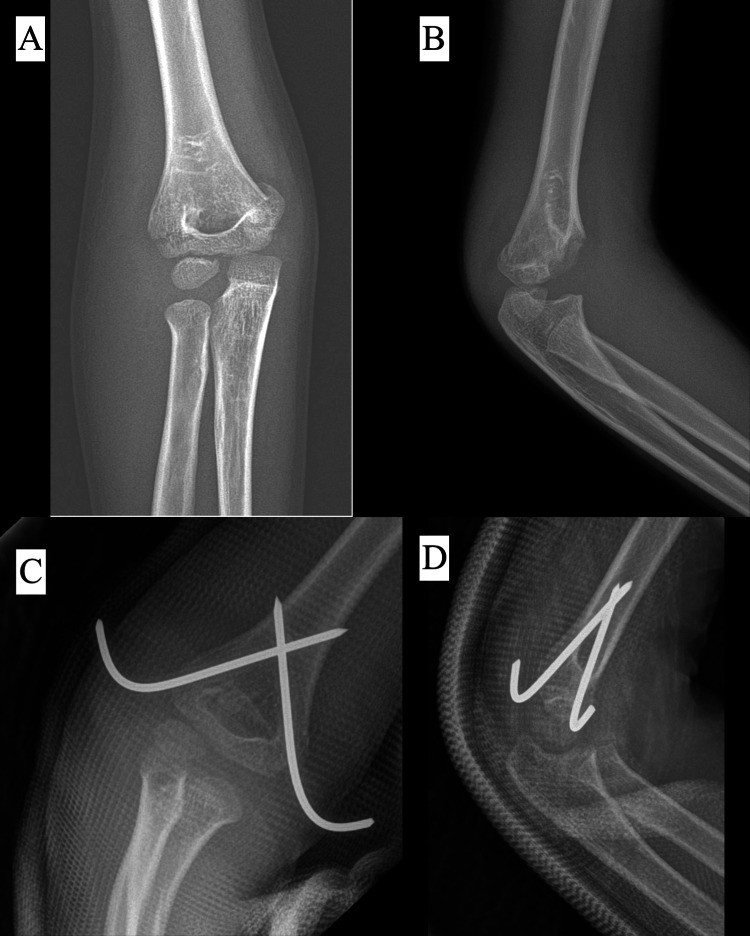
Radiograph of a five-year-old patient with a Type II supracondylar fracture (A) Preoperative AP view. (B) Preoperative lateral view. (C) Postoperative AP view showing crossed pin configuration. (D) Postoperative lateral view.
